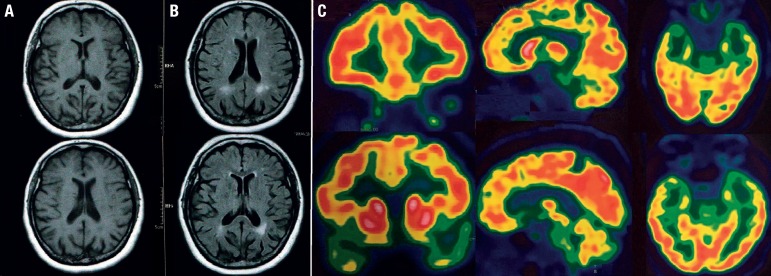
A. Magnetic resonance imaging (MRI) of brain showing moderate parietal atrophy and no focal frontotemporal atrophy. B. Moderate white matter disease on brain MRI. C. Positron emission tomography with fluorodeoxyglucose (PET-FDG) showing marked hypometabolism in temporal poles and anterior cingulate; there is mild hypometabolism in the orbitofrontal cortex, medial temporal regions (including hippocampus) and temporo-parietal cortex. No hypometabolism is evident in the precuneus and posterior cingulate.
